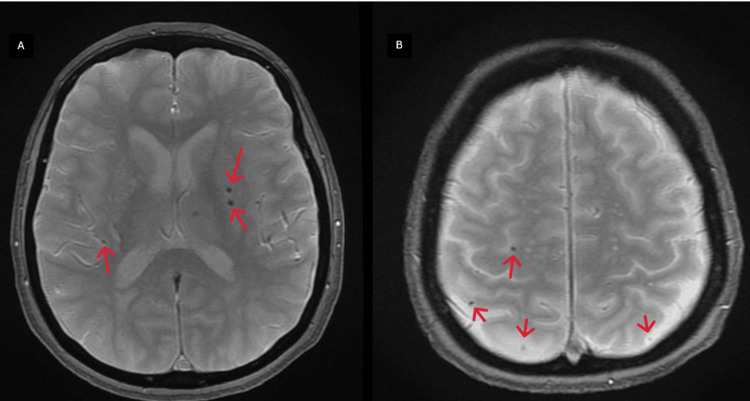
Axial view of cerebral MRI (T2*). (A,B) The bilateral microhemorrhages at different levels (red arrows) MRI: magnetic resonance imaging
